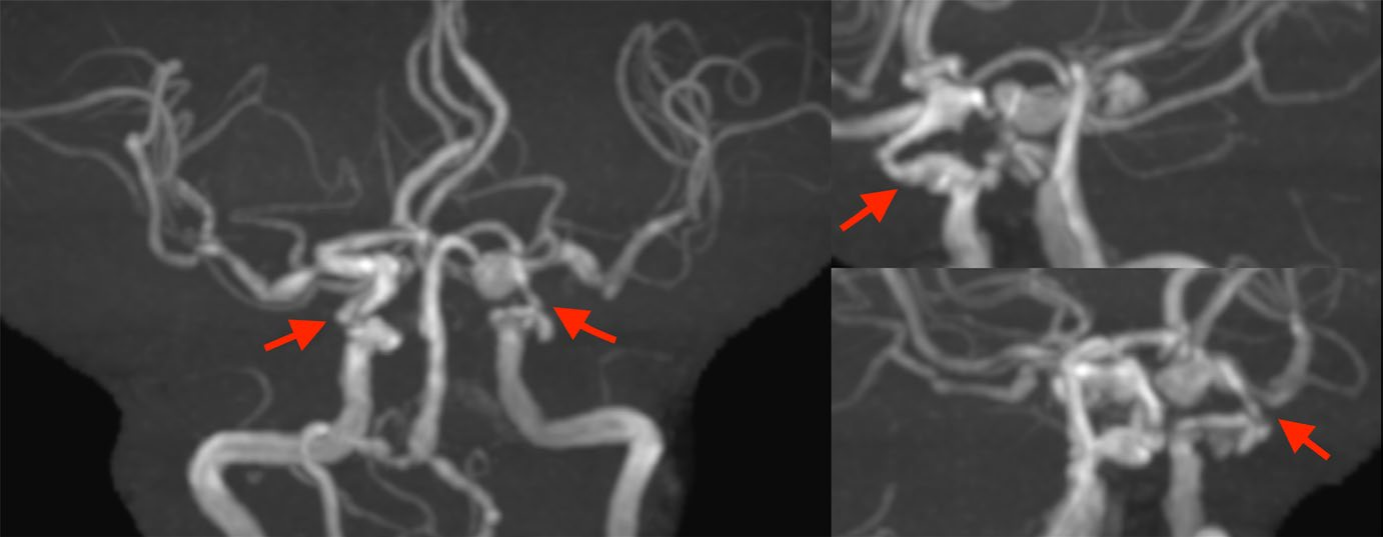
Brain magnetic resonance imaging revealed multiple stenosis of the cerebral arteries, mainly in the bilateral internal carotid artery siphon site (arrow)
